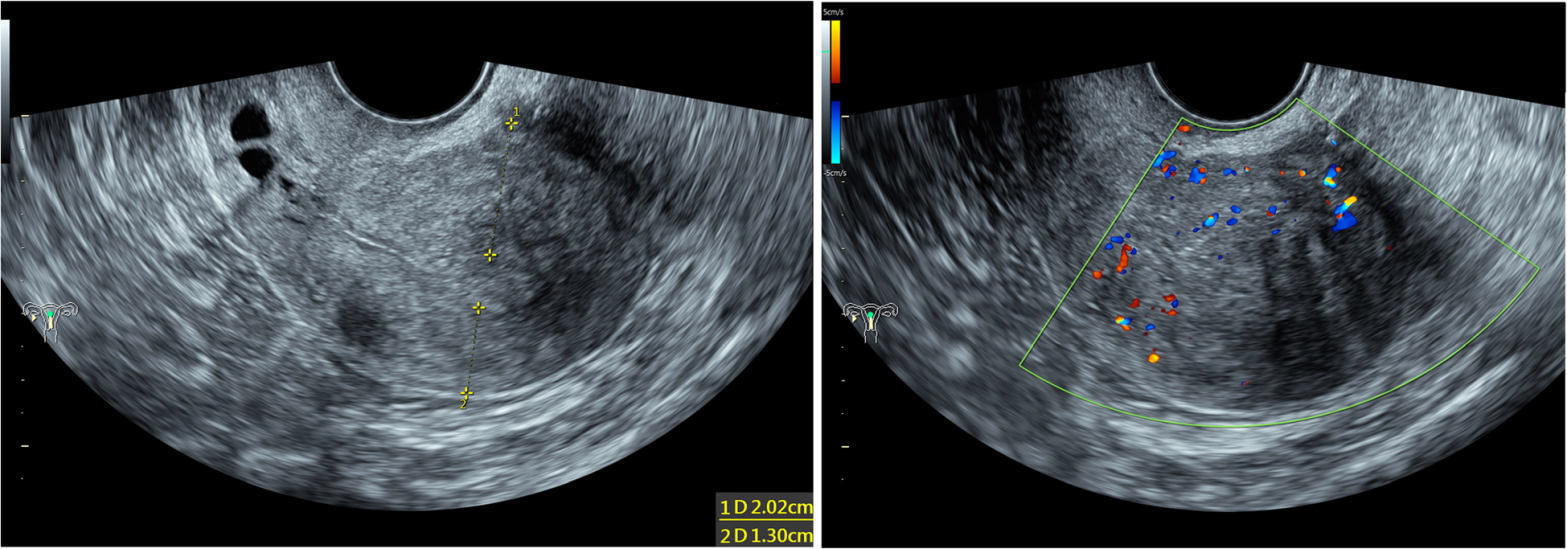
Transvaginal ultrasonography (TVS) of uterus with adenomyosis . TVS of Case 1 shows enlarged uterus, with posterior uterine wall thickening and hypoechoic linear myometrial striations into the myometrium
